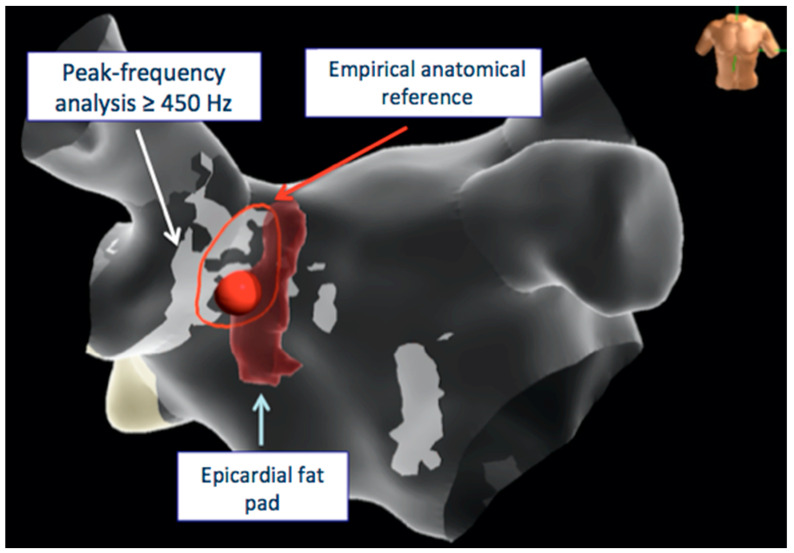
Multimodal integration of GP localization strategies: convergence of empirical anatomical reference, CT-segmented epicardial fat pads (ADAS 3D), and peak-frequency analysis (OTNF ≥ 450 Hz) on an electroanatomic map (EnSite X).
